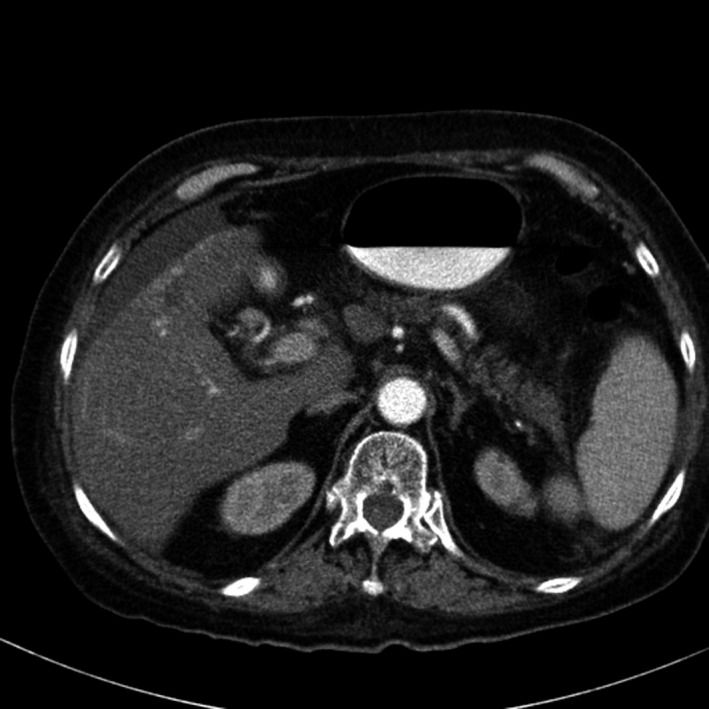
Patient 2. 2.5‐month follow‐up CT image arterial phase, the maximal tumor diameter was 2.9 x 2.3 cm, this patient had worsening complications of cirrhosis as well as can be seen by evolving ascites although the tumor was showing some response.
